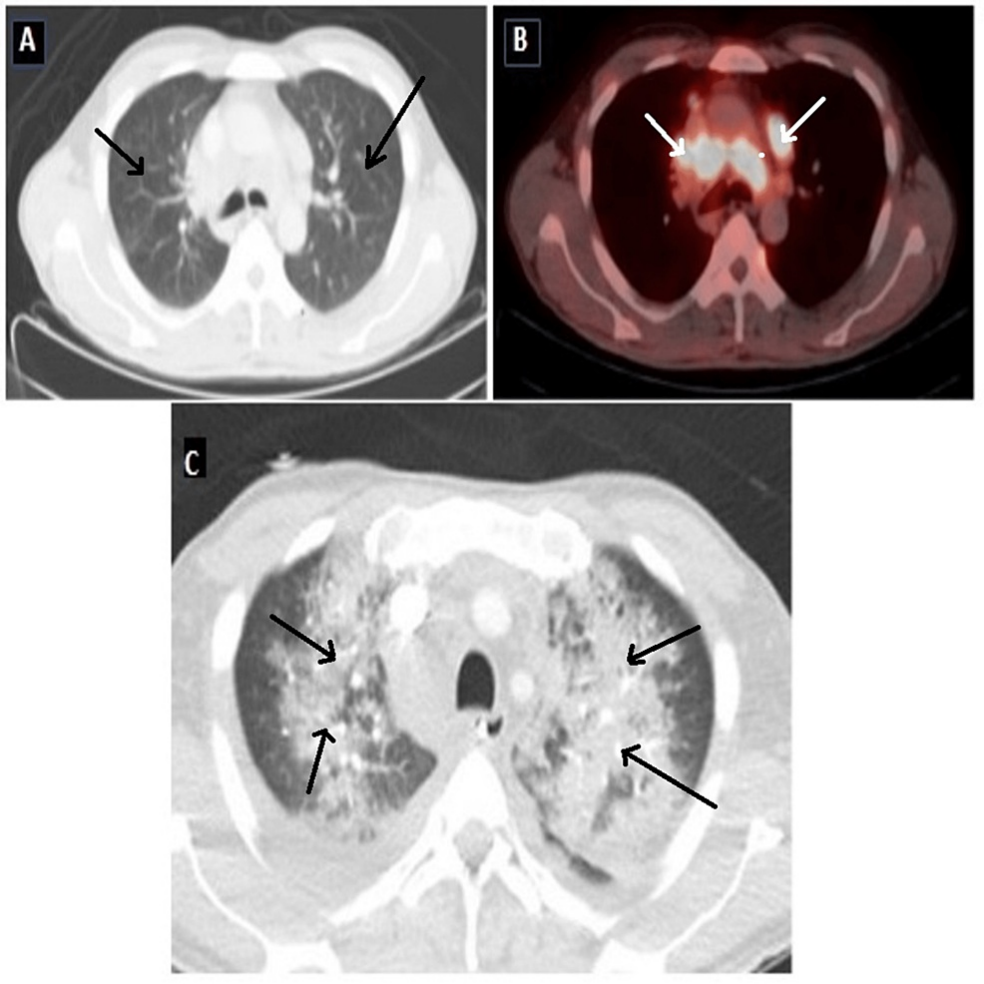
Baseline F18-FDG PET-CT scan Axial images of the CT (A) and Fusion PET-CT (B) at the level of tracheal bifurcation shows normal lung parenchyma and hypermetabolic mediastinal lymphadenopathy respectively. ​The CT scan (C) through the chest shows bilateral patchy lung infiltrates suggestive of bleomycin-induced lung injury. PET-CT: Positron emission tomography-computed tomography, F18-FDG: Fludeoxyglucose F18
